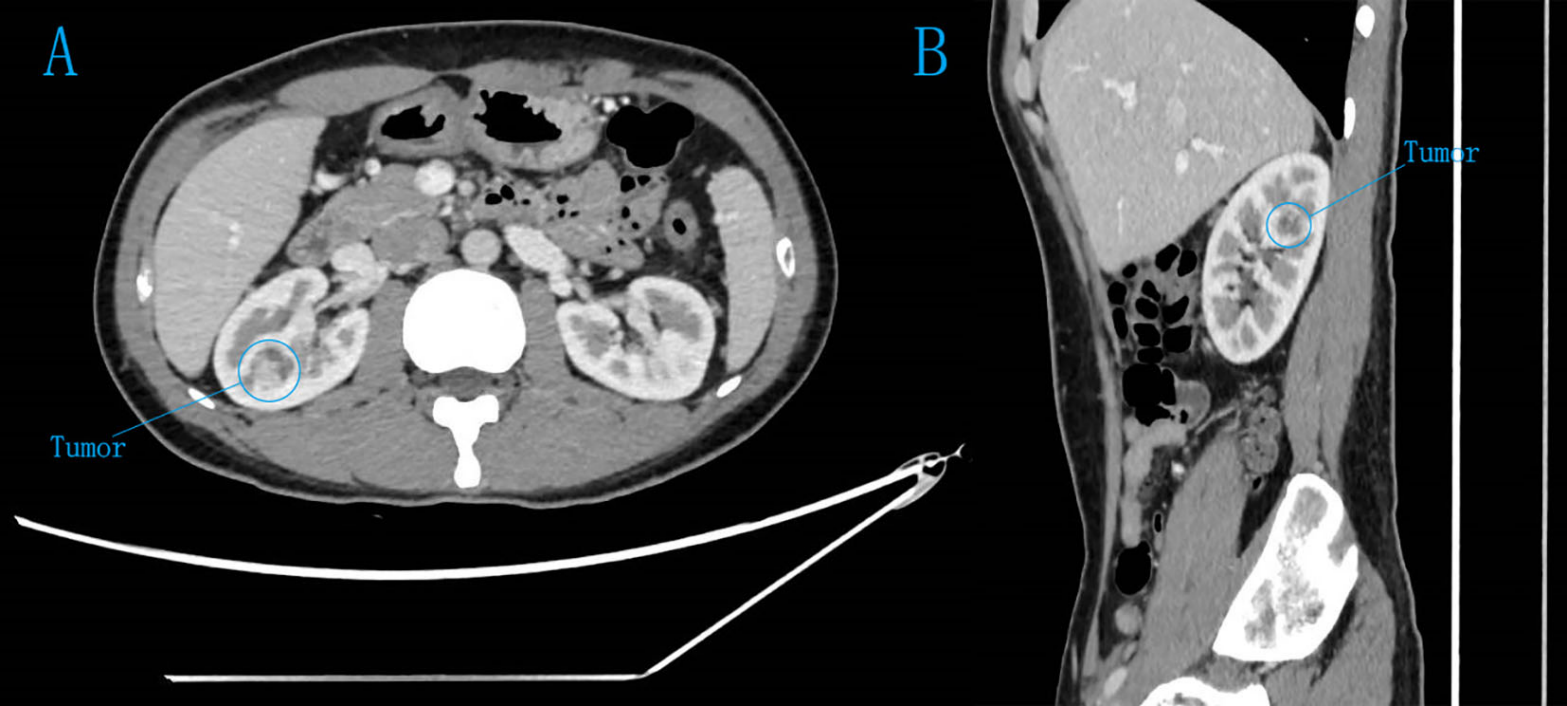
CT plain scan and enhanced images of completely endogenous renal tumours. (A) axial view. (B) Sagittal view. Contrast-enhanced CT scan of both kidneys in the cortical phase reveals a markedly heterogeneous enhancing mass in the right kidney.
